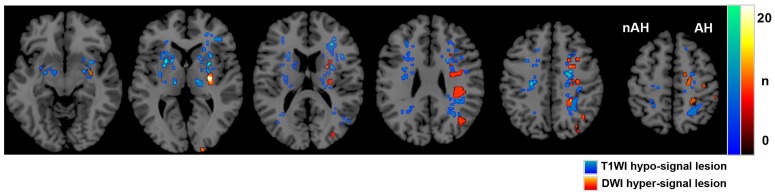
MRI lesion distribution of 37 patients using SPM. This image shows the lesion distribution on MRI of the 37 patients included in the present study. The numbers of patients with ischemic lesions are shown by color-coding. Lesions that were hypointense on T1WI are colored blue to green, whereas lesions that were hyperintense on DWI are colored red to yellow. The lesions are seen predominantly in the affected hemisphere, and the distributions show the watershed type of infarction. The images were anatomically standardized by SPM. AH: affected hemisphere, nAH: non-affected hemisphere.
